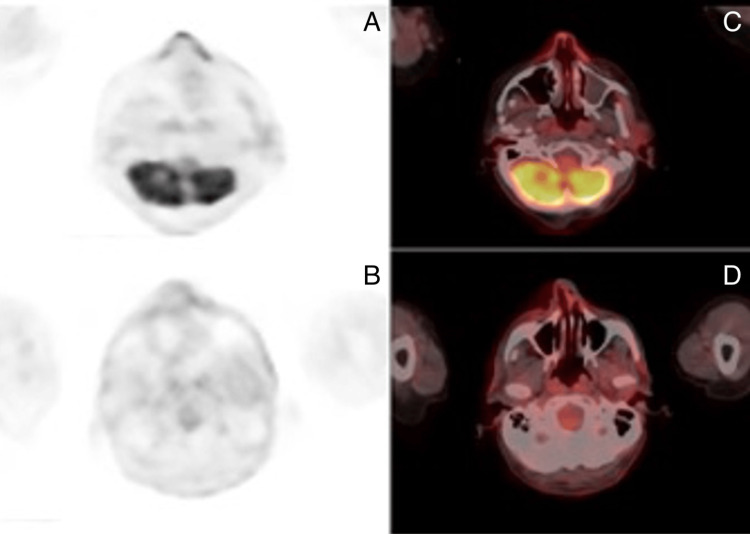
The PET CT image of tubarial glands: MIP (right) (A, B) and axial fused images (left) (C, D) of 18F-FDG PET/CT PET CT: Positron emission tomography-computed tomography, MIP: Maximum intensity projection, FDG: Fluorodeoxyglucose
